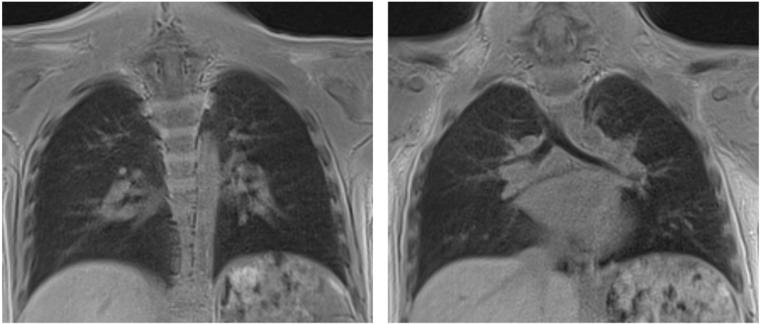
Ultrashort echo time (UTE) MRI sequence of the chest in a 6-year-old boy, demonstrating detailed visualization of the lung parenchyma.
